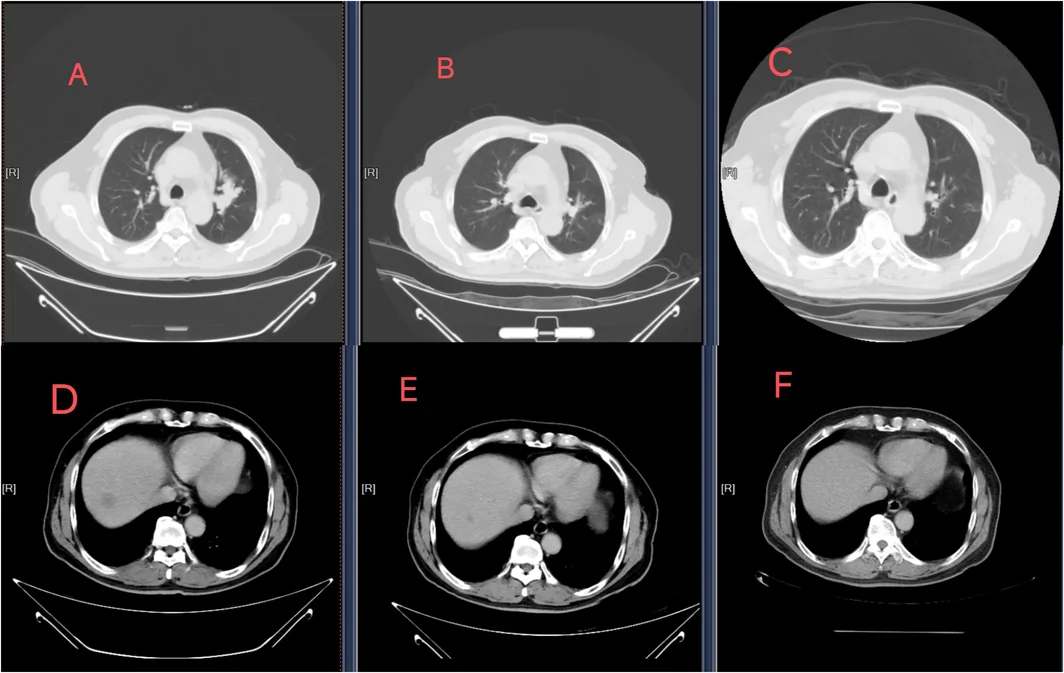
Imaging comparison of target lesions before and after treatment. (A) Baseline CT image of primary lung lesion (August 2024); (B) Lung lesion after 2 cycles of combined treatment; (C) Lung lesion after 11 cycles of tislelizumab‐based treatment; (D) Baseline CT image of liver metastatic lesions; (E) Liver metastatic lesions after 2 cycles of combined treatment; (F) Liver metastatic lesions after 11 cycles of tislelizumab‐based treatment. All lesions achieved complete regression at the final follow‐up.
